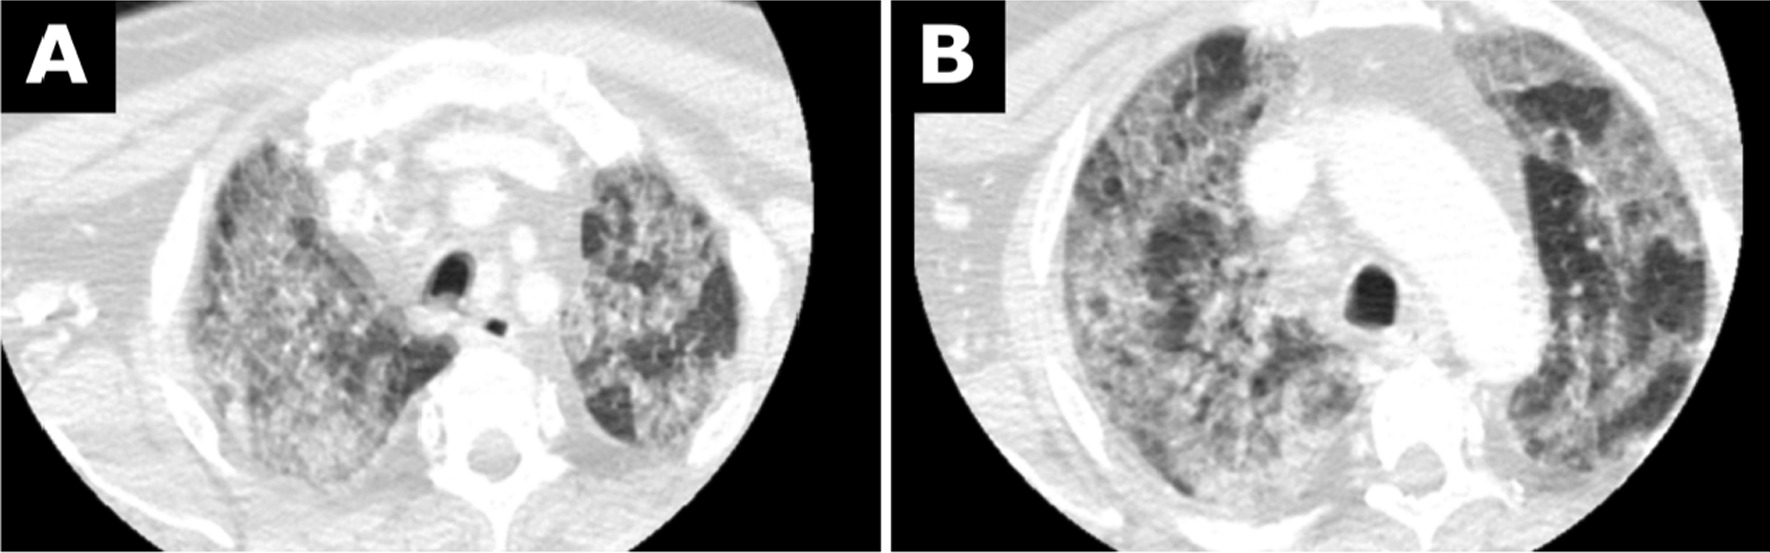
A, B Chest computed tomography images as part of head and neck computed tomography from 3 January 2020
